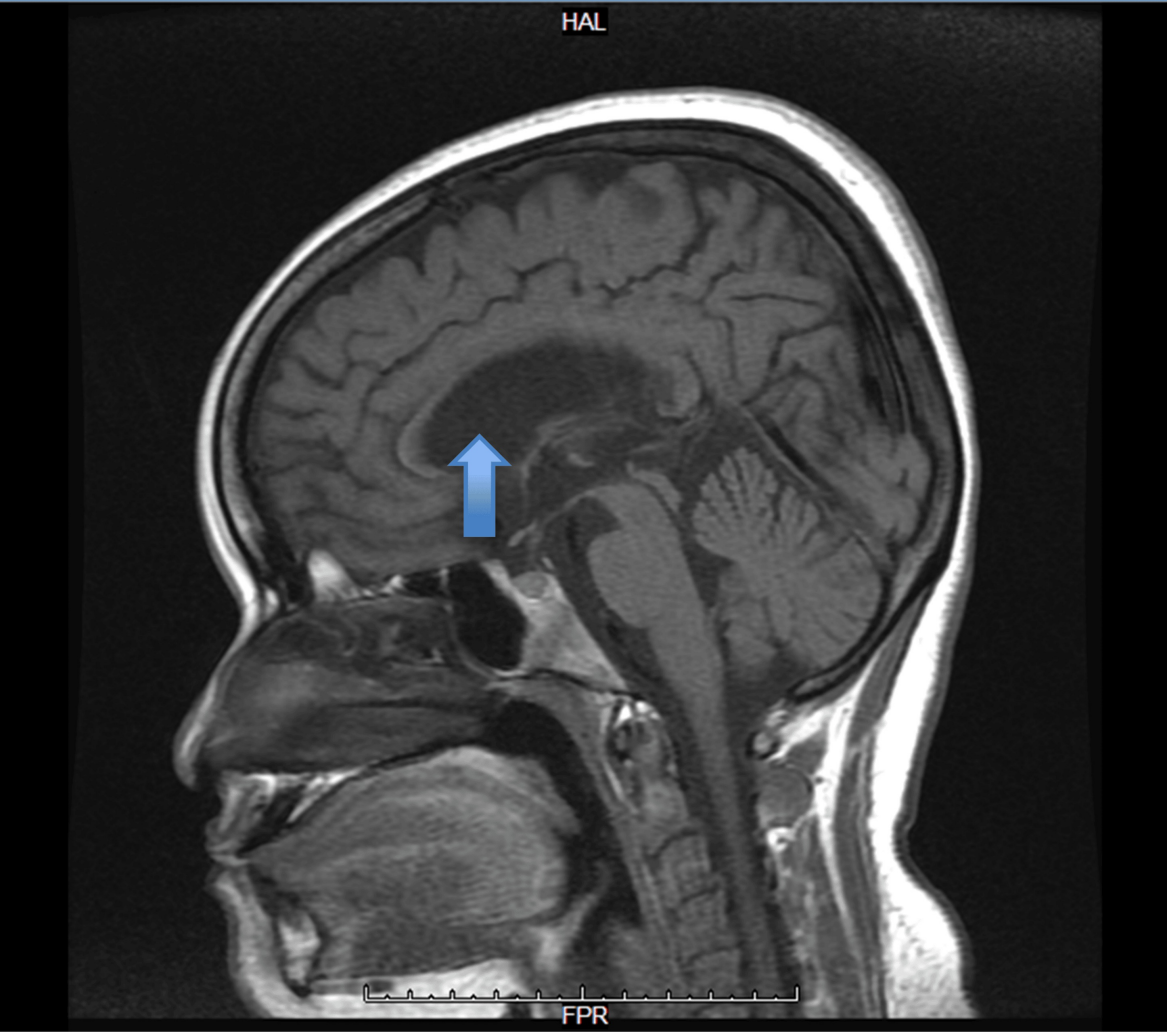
Sagittal T1-weighted MRI of the brain Sagittal T1-weighted MRI demonstrates diffuse cortical atrophy with enlarged ventricles (blue arrow) disproportionate to age. The midline structures, including the corpus callosum, brainstem, and cerebellum, are preserved. No midline shift, mass effect, or abnormal enhancement is present MRI: magnetic resonance imaging
